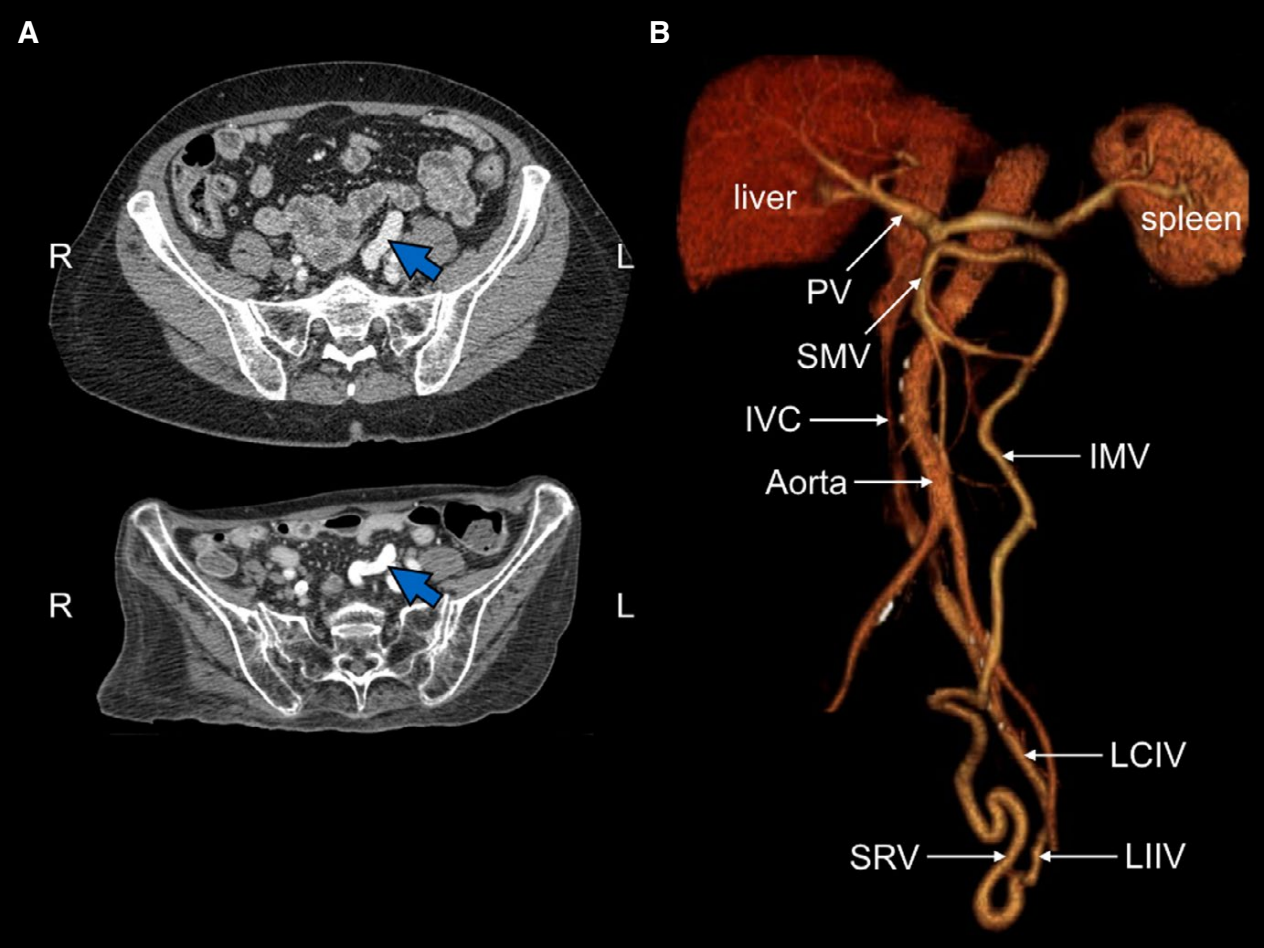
Imaging of portosystemic shunt. A, CT scans. Blue arrow indicates dilated superior rectal vein, present from 6 months prior to first hospital admission (upper panel) to 8 years later (lower panel). Note dramatic weight loss. B, CT reconstruction of vascular system. Note shunt of superior rectal vein (SRV) to the left internal iliac vein (LIIV). The shunt produces early opacification of the left common iliac vein (LCIV), but not the right common iliac vein. Other abbreviations: IVC, inferior vena cava; IMV, inferior mesenteric vein, PV, portal vein; SMV, superior mesenteric vein
